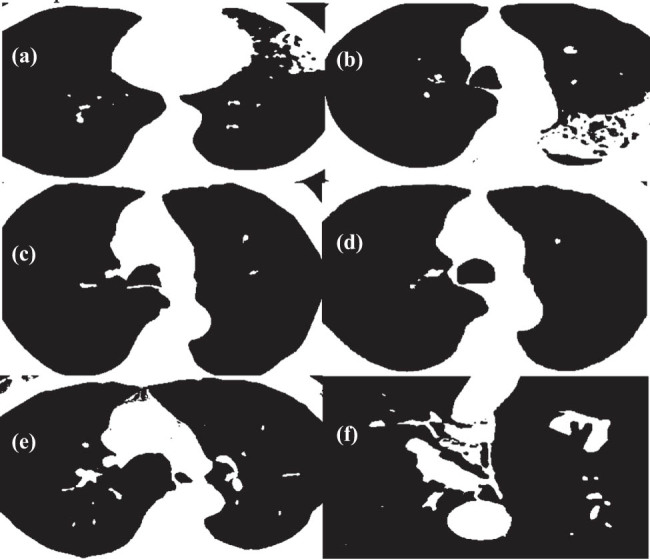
A 55-year-old male patient with intermittent cough and asthma for 5 years, aggravated with bloody sputum, and high fever for 11 days. Ten days before admission, the patient developed bloody phlegm without obvious inducements and had pain in the left anterior thoracic region. (a) and (b) Initial CT images at admission; (b) and (c) CT images during the treatment; and (d)–(f) re-examined CT images 2 months after the treatment.
